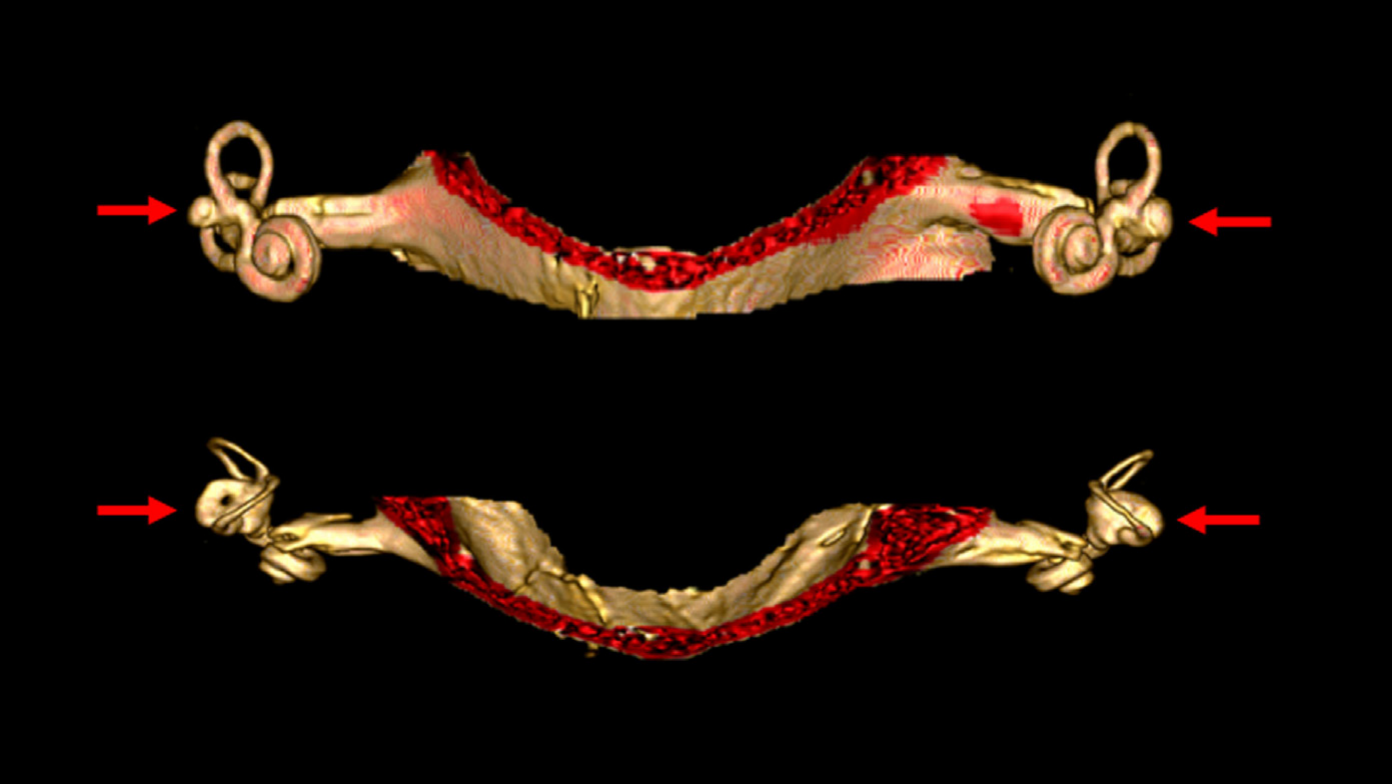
High resolution three-dimensional reconstruction MRI of the internal auditory canals and inner ear structures of a patient with bilateral vestibular weakness from bilateral labyrinthine dysplasia. The top image is in the coronal aspect. The bottom image is in the axial aspect. In these images it is evident that the horizontal canals are dysplastic, with the horizontal canal and vestibule appearing as a single abnormal structure on each side (indicated by the arrows). The superior and inferior canals are present as true canals, but are somewhat hypoplastic.
